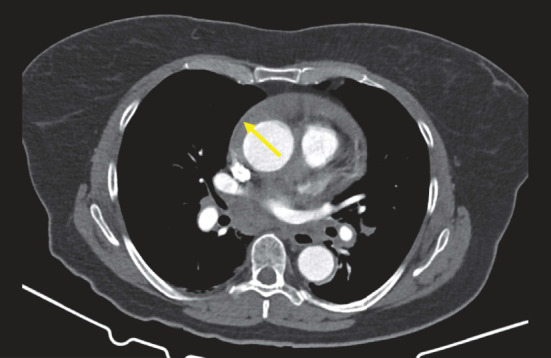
Contrast-enhanced computed tomography of the aorta, performed at the referral hospital on a slice corresponding to Figure 3, shows a contrast-filled aorta without visible flaps and with a normal diameter. However, a subtle delineation between the aortic wall hematoma and the pericardial effusion remains visible (indicated by an arrow).
